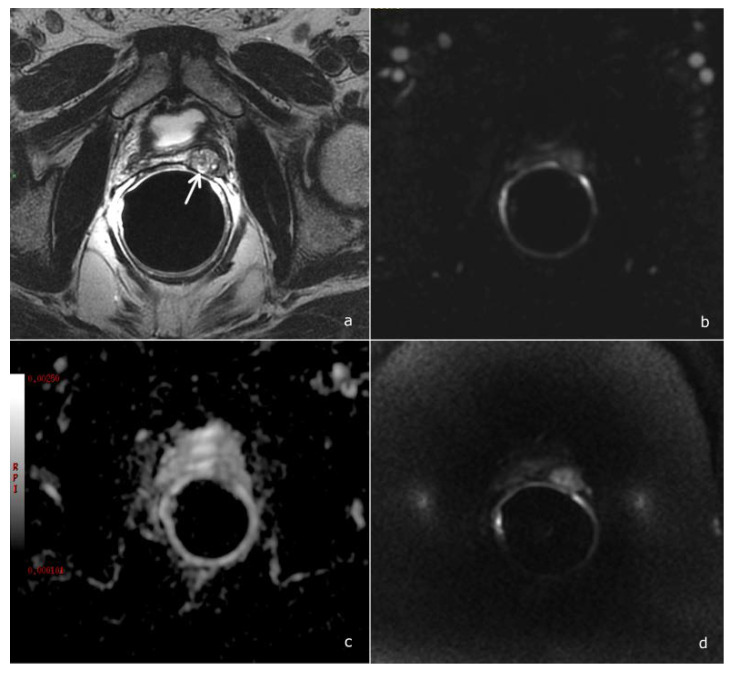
A PI-RR-score-2 patient, 68 years old, with a PSA value of 0.45 ng/mL. (a) T2-weighted image shows a hyperintense tissue in the rectovesical region (arrow), characterized by a homogeneous and diffuse contrast enhancement, (b) non-pathological signal abnormality on the ADC map, (c) slightly hyperintensity on DWI, (d) a TRUS-guided biopsy was performed, documenting benign prostatic tissue remnants.
